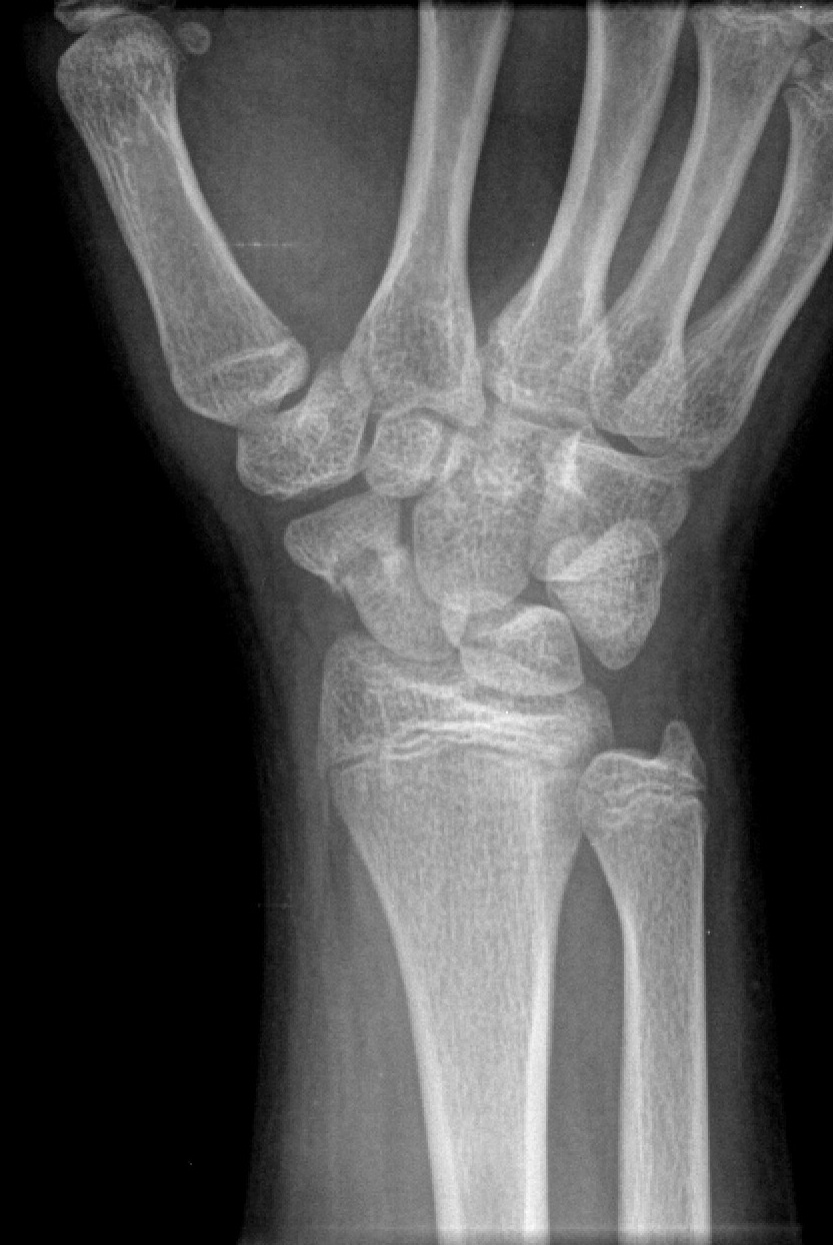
Radiograph of Case #3. Fracture through scaphoid located just distal to waist with mild displacement. Slight widening of the scapholunate interval is also noted. No avascular necrosis is evident.
